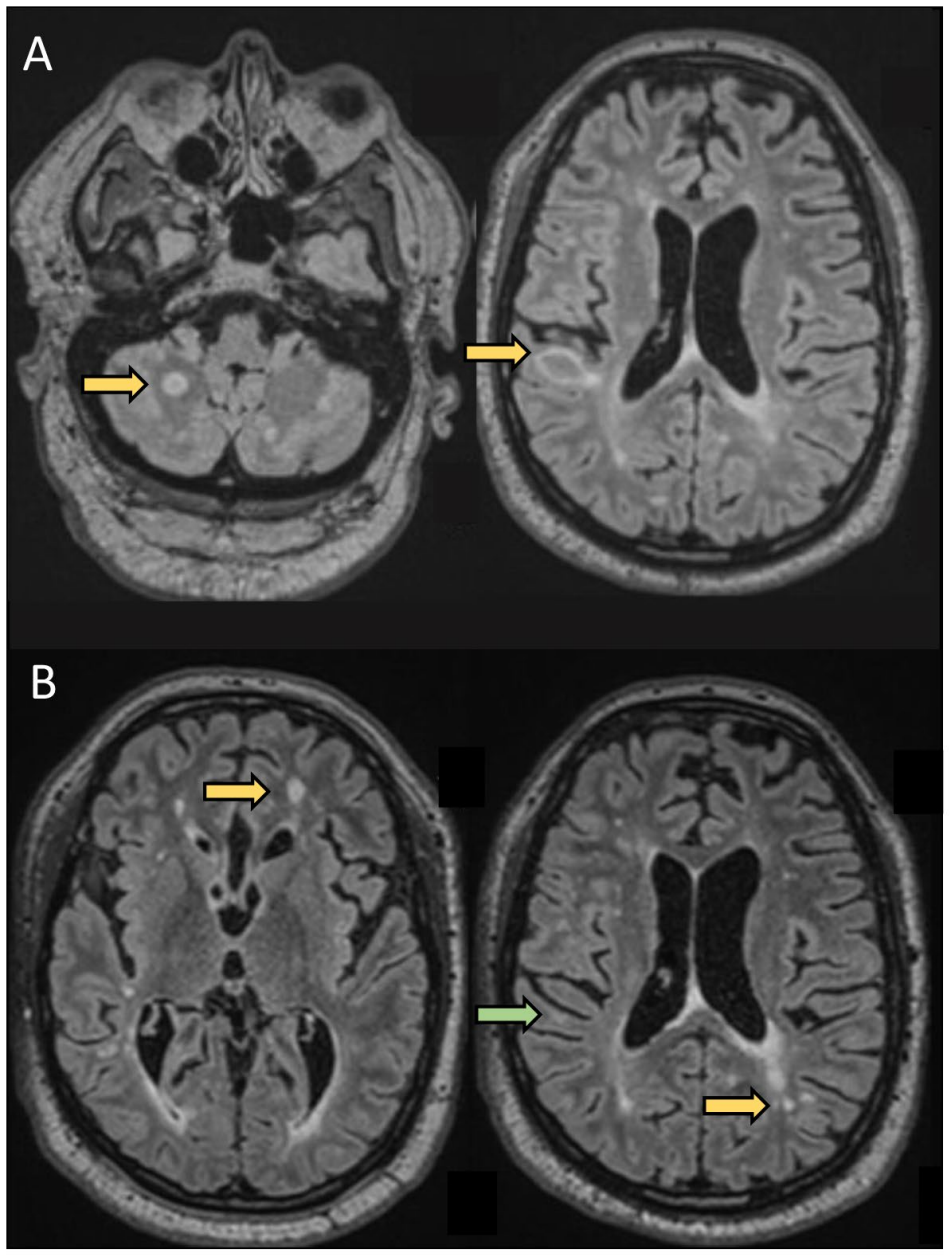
A 61-year-old man was evaluated for new neurological symptoms suggestive of a relapse. He was diagnosed with RRMS in 1998 after two episodes compatible with sub-acute sensory myelitis in 1994 and 1998. He did not receive any treatment for his MS between 1998 and 2019. Between 2000 and 2008, he had a couple of episodes suggestive of mild relapses. He had also noticed some progressively worsening gait instability and cognitive difficulties over the past few years, In April 2019 (at the age of 57), he experienced transient tingling followed by mild weakness and ataxia of the left upper extremity which resolved spontaneously over 5 weeks. Brain MRI showed 2 new lesions, one in the right juxtacortical posterior frontal lobe in the precentral gyrus explaining his symptoms. He was started on dimethyl fumarate 240 mg BID. He was doing well until August 2020 (at the age of 58 years), when he experienced tingling in the RUE associated with worsening cognitive difficulties. Repeat MRI showed 2 new lesions in the supratentorial regions. He was switched to oral cladribine without new clinical events or new radiological activity. *This case highlights that although rare, some patients have continued disease activity despite an older age and longer disease duration*.(A) Axial FLAIR sequences of brain MRI in April 2019 showing 2 new lesions (arrows) compared to prior MRI in 2017, and concomitant with a relapse (weakness and proprioceptive ataxia of the left upper extremity). (B) Axial FLAIR sequences of brain MRI in August 2020, 13 months after starting dimethyl fumarate, showing 2 new lesions (yellow arrows) compared to MRI in April 2019, with a decrease in size of the right posterior frontal lesion seen on prior MRI (green arrow) concomitant to a relapse: weakness of LUE and LLE.
